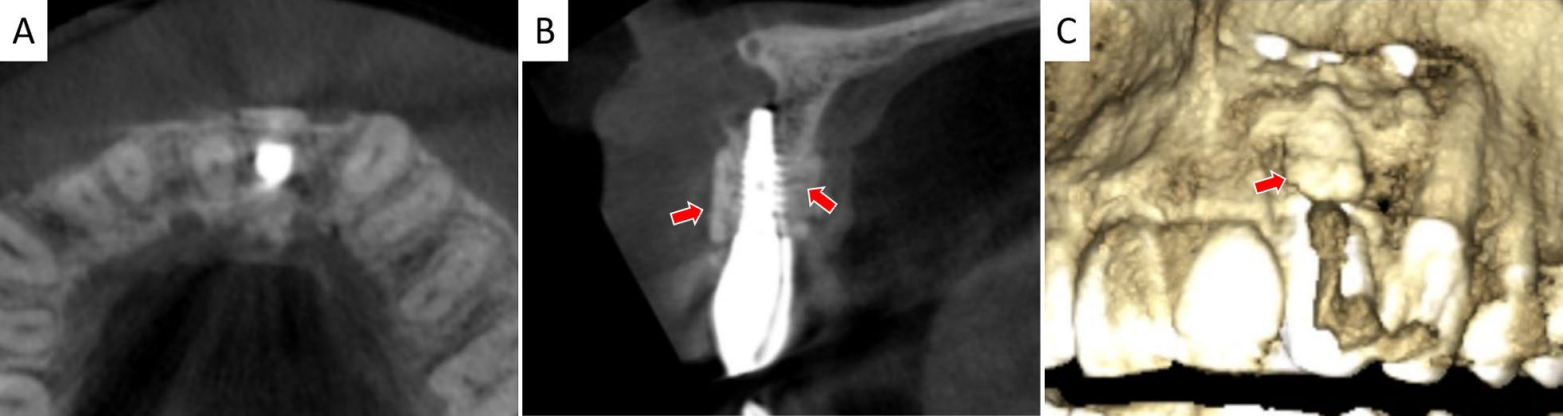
CBCT examination 1 year after implant-supported prosthesis. A–C showed coronal-, sagittal plane and 3D reconstruction views. Red arrows depict TS still existed
